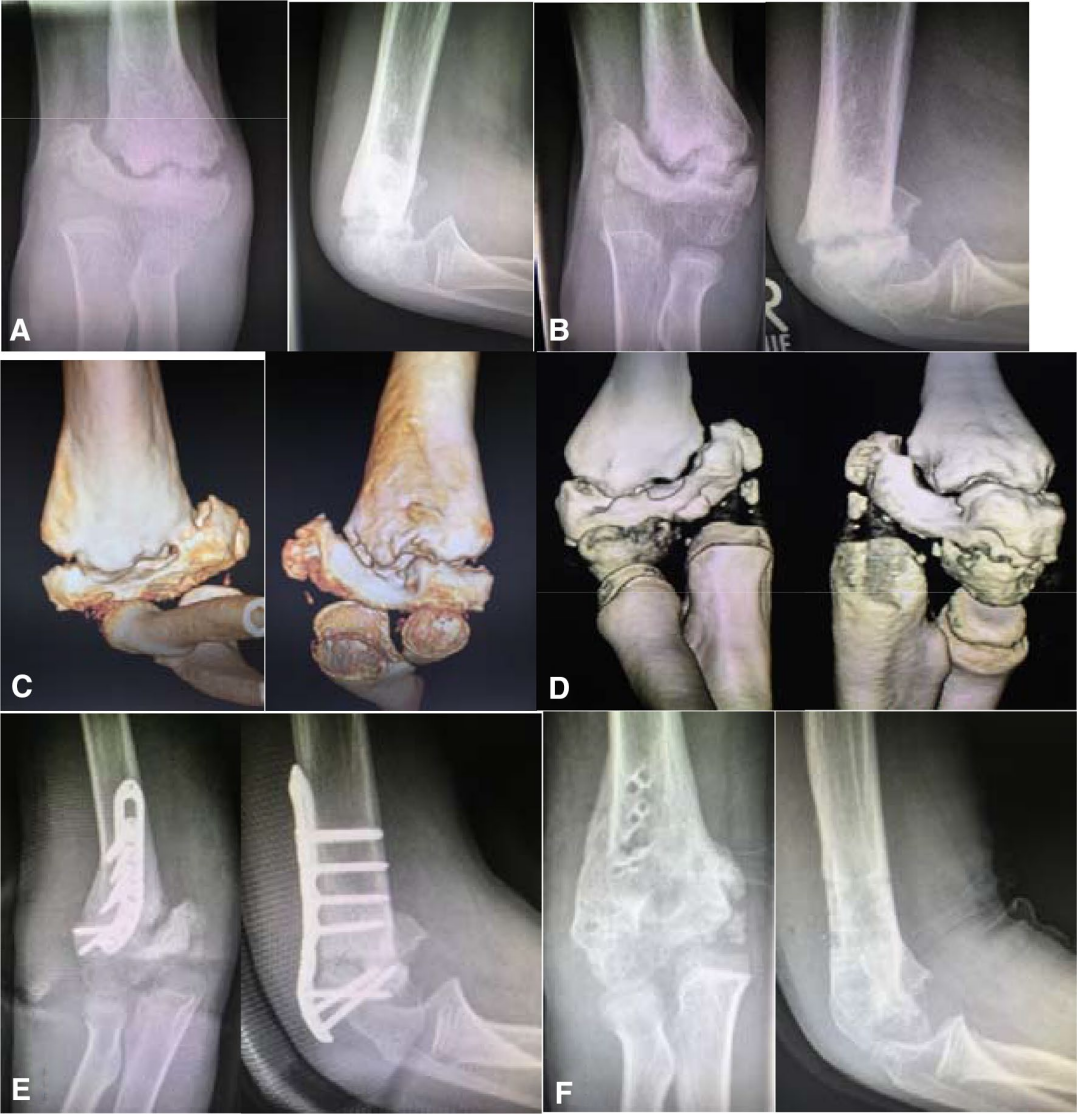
a Images after 6 and b after 12 months. c 3D-CT after 12 and d after 18 months. e Postoperative control after radial distal humerus plating via triceps-sparing approach and cancellous autograft. f After implant removal 1 year after plating and bone-grafting
